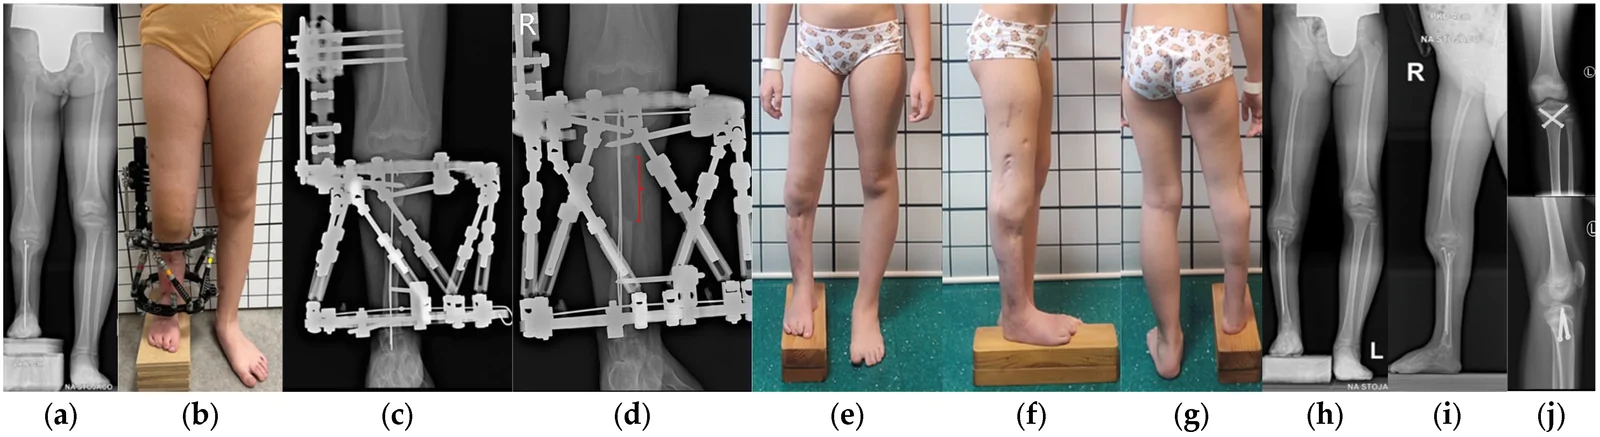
Fibular lengthening procedure: (a) preoperative appearance; (b) clinical view during the first 6 cm fibular lengthening using an external fixator; (c,d) radiographic view during lengthening; (e–g) clinical result; (h,i) radiographic result; (j) growth arrest of the contralateral tibia. NA STOJĄCO—standing position, L—left, R—right, red curly brace—bone regenerate.
